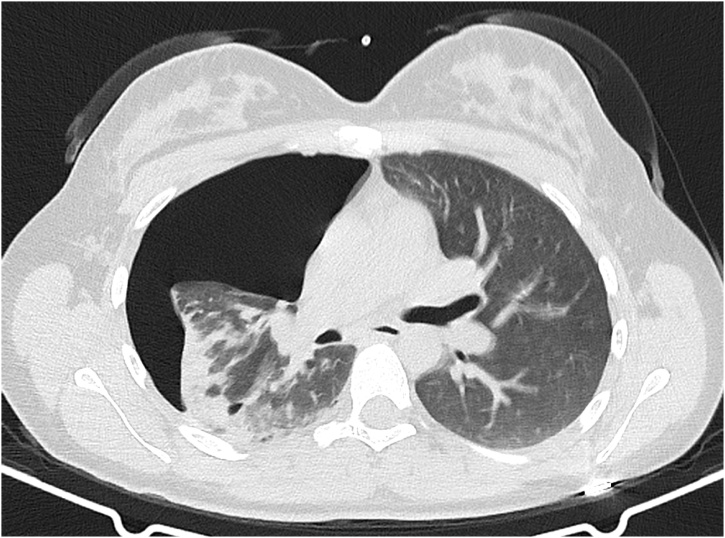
Thoracic computed tomography showing pneumothorax.
